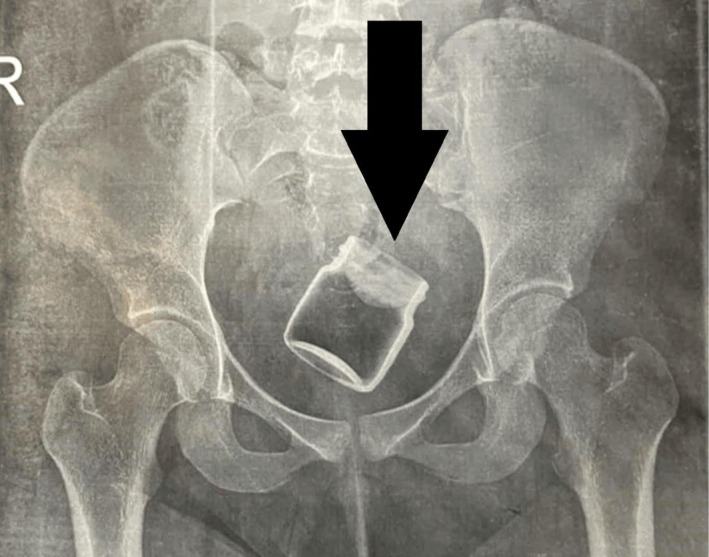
Plain radiograph revealing a well‐defined, radiopaque object consistent with a jam jar situated in the mid‐pelvic cavity.
